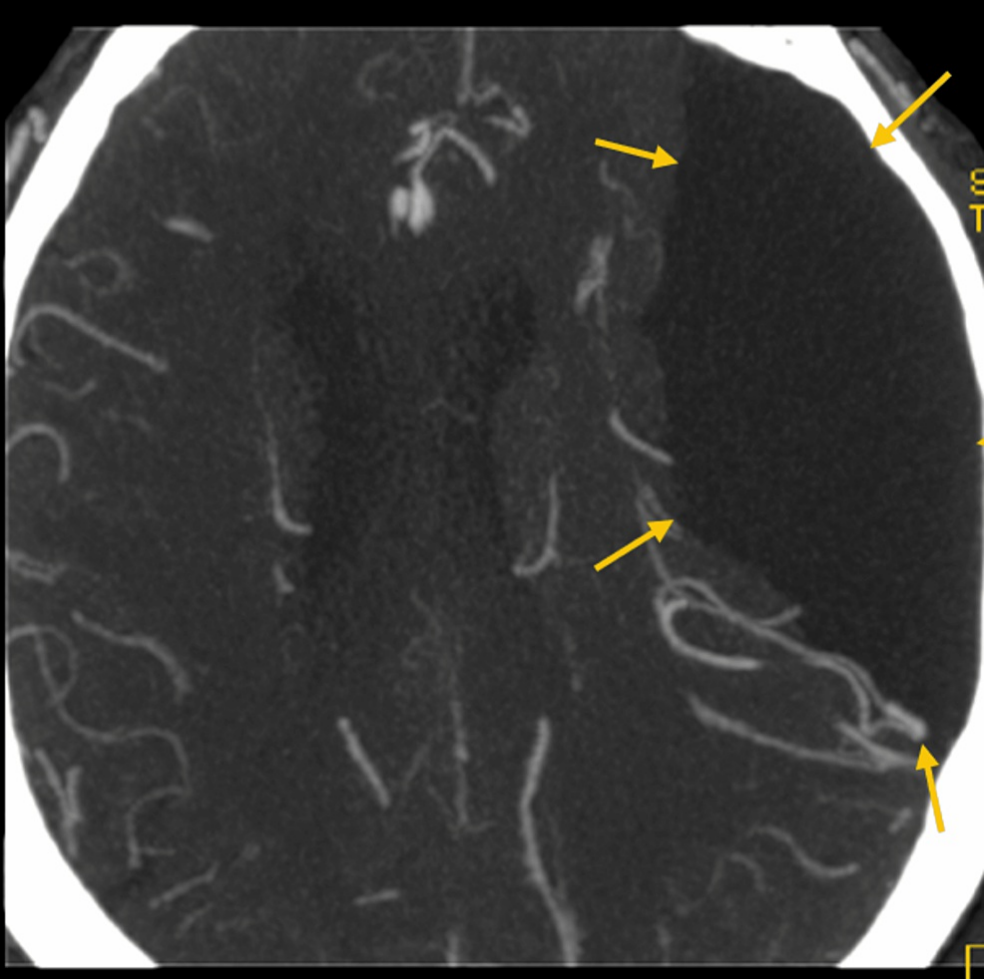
Brain computed tomography with angiography Brain computed tomography with angiography in axial view showing the large extra-axial fluid collection and its compressing effect on the brain parenchyma and vasculature.
